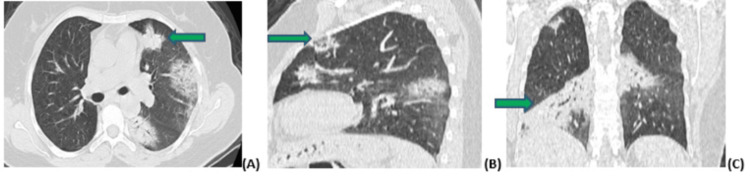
Figure 3 (A-C): Axial, sagittal, and coronal CT sections showing foci of pulmonary condensation marked at the level of the left superior lobe (green arrow), forming part of chronic eosinophilic pneumonia
